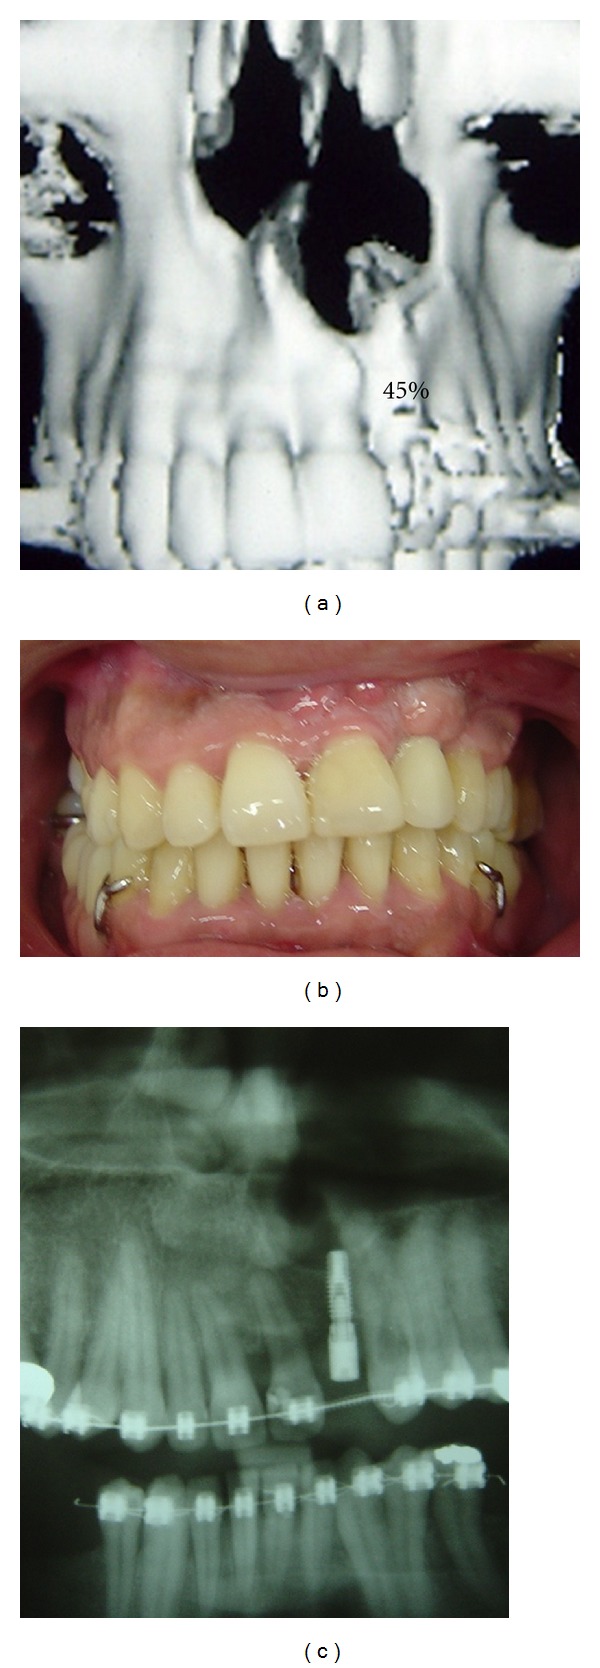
Patient with grade II. (a) CT scan view; (b) occlusal view; (c) panorex demonstrating dental implant.
